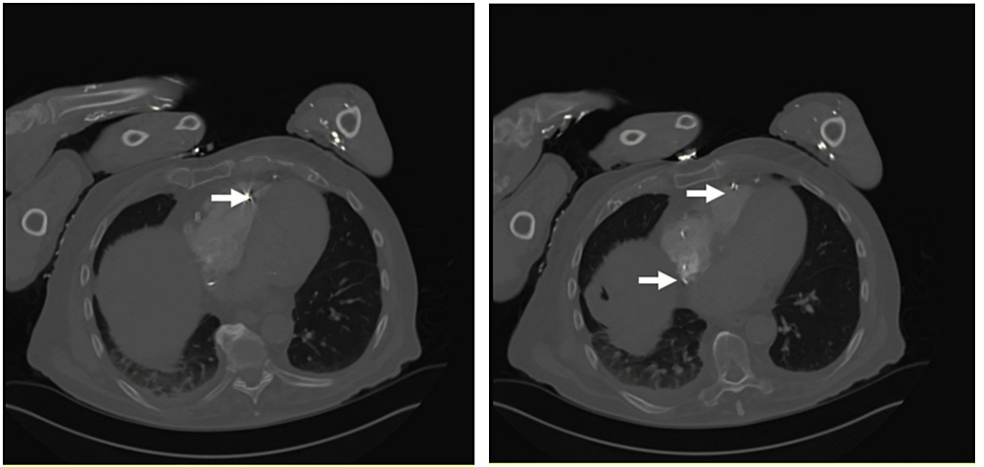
A panel of CT images demonstrates hyper-attenuating structures within the right lower lobe cavity, suggestive of gallstones. CT: computerized tomography
